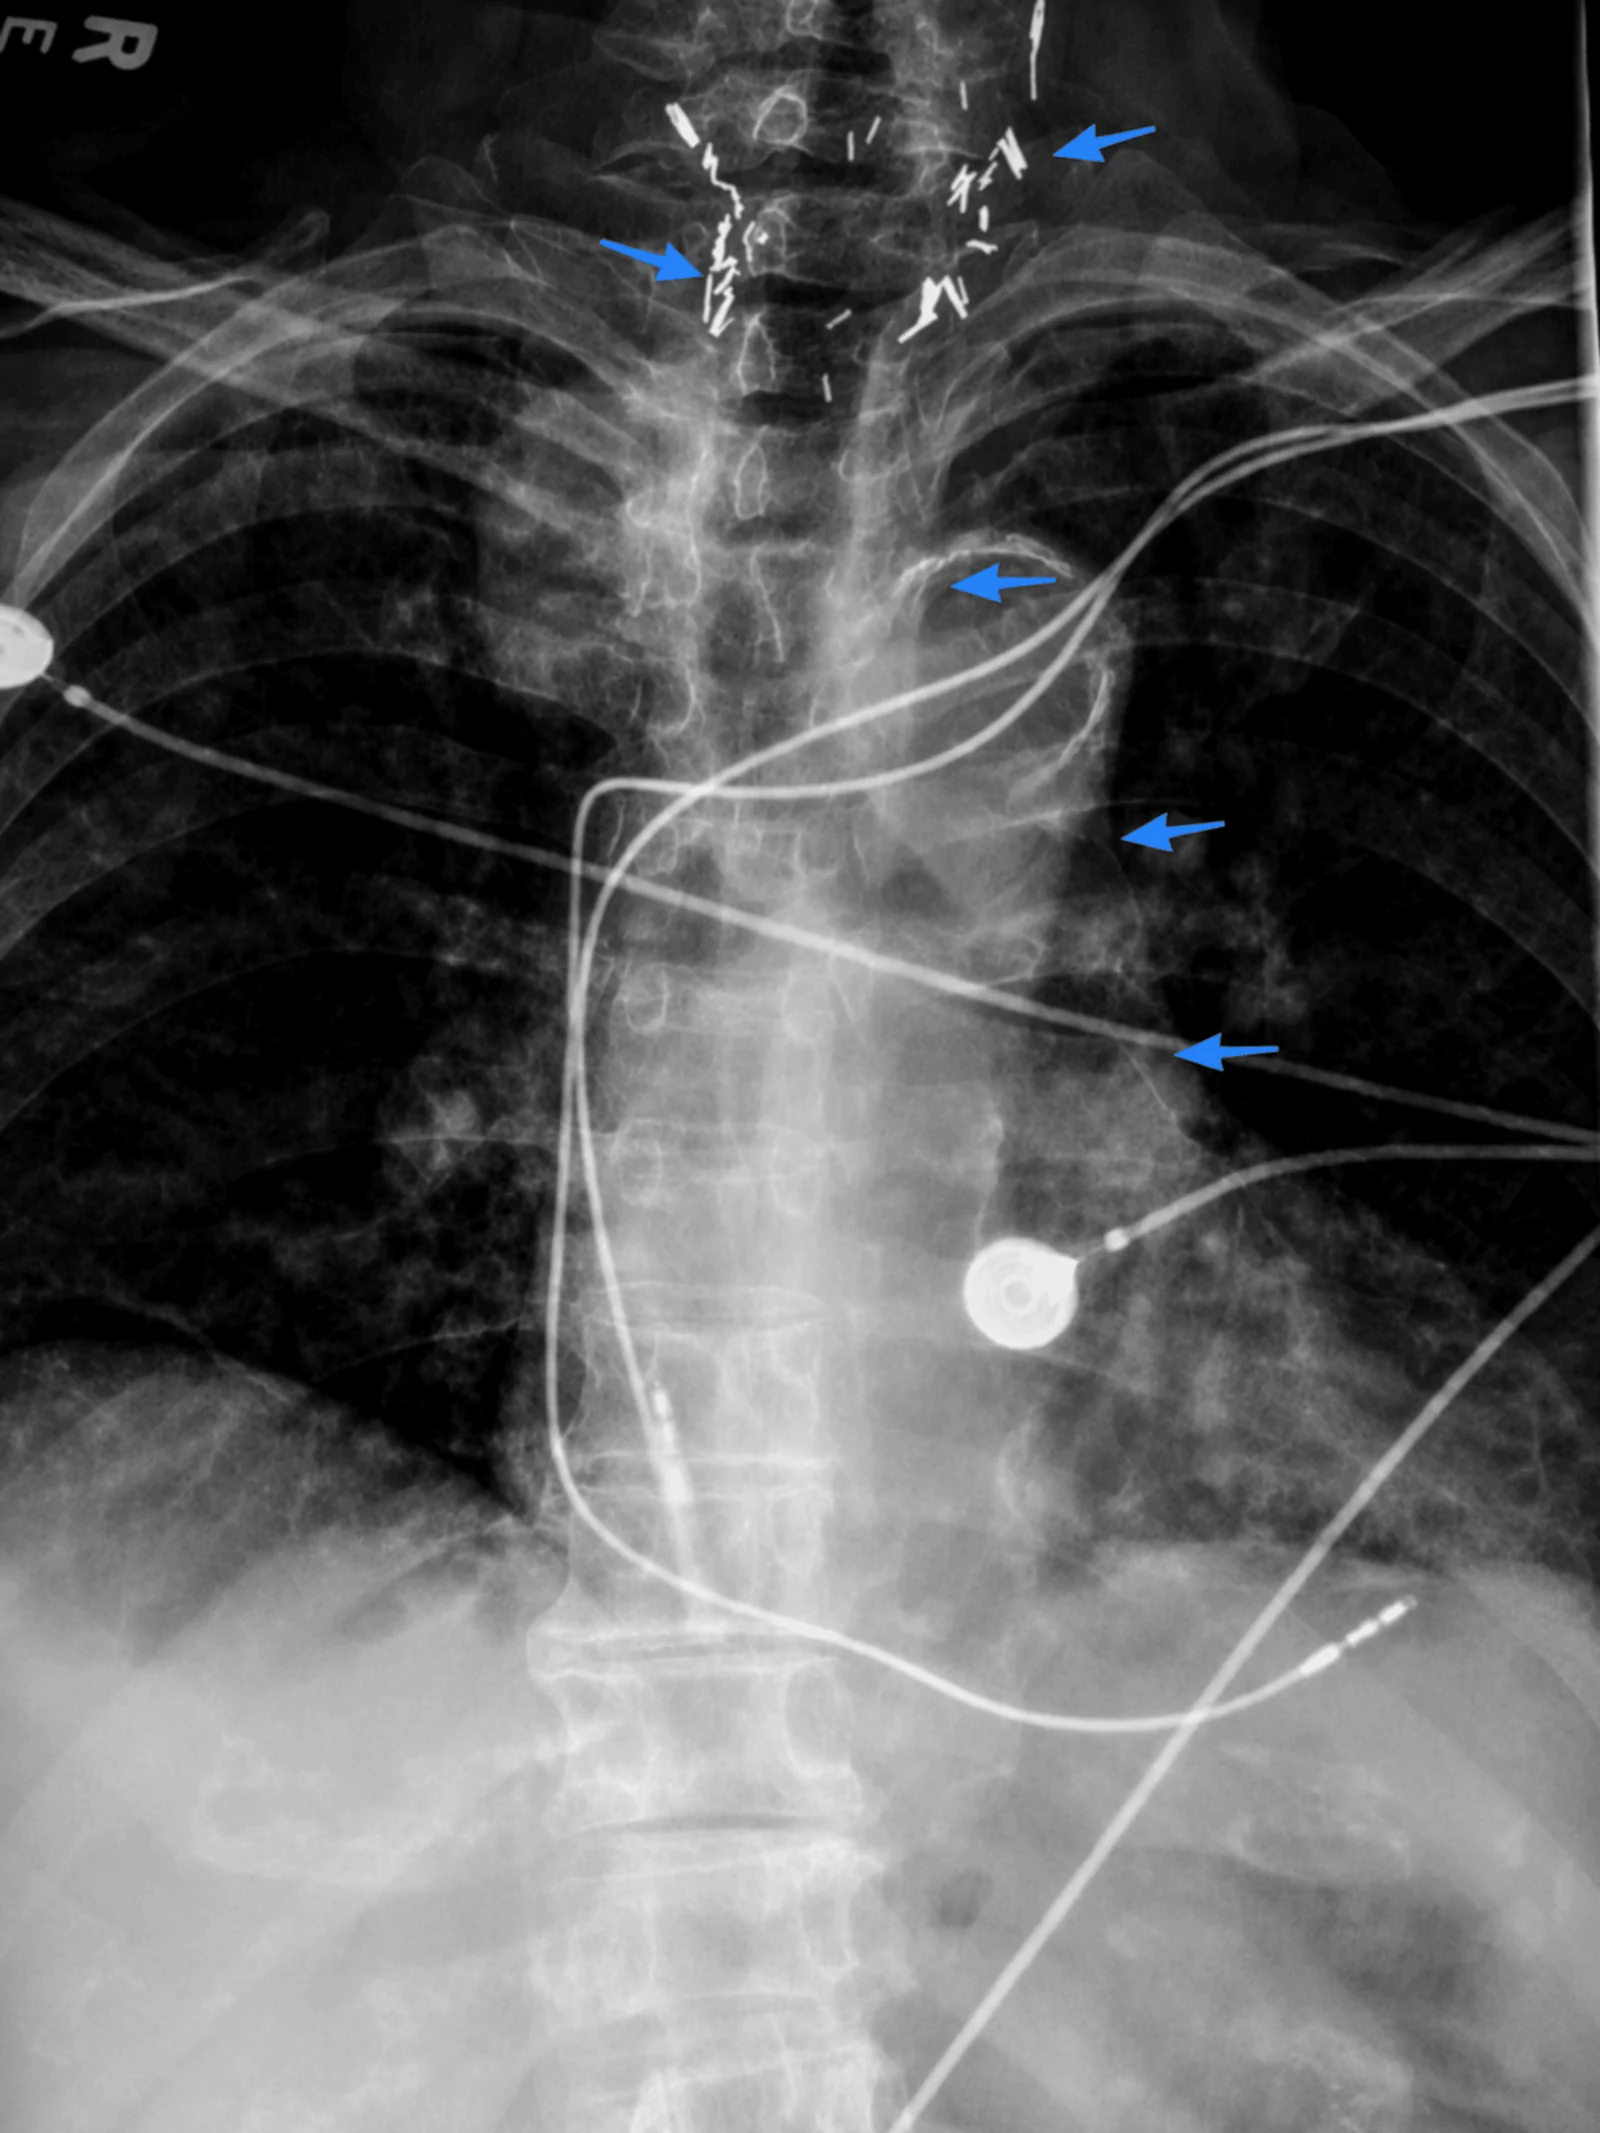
X-ray of the Thoracic Spine X-ray of the thoracic spine showing no significant abnormality of the thoracic spine and severe aortic calcifications (blue arrows).
